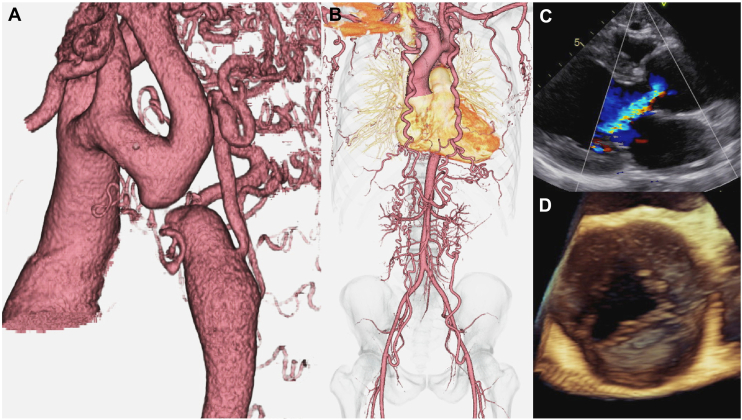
( A) Three-dimensional computed tomography shows loss of continuity of the aorta distal to the left subclavian artery bifurcation and ductus arteriosus and notably developed collateral circulation. (B) The brachial and left subclavian arteries are notably developed, with other collateral blood vessels to the lower limbs via the inferior abdominal wall. (C) Preoperative transthoracic echocardiography shows aortic root enlargement and severe aortic regurgitation. (D) Bicuspid aortic valve with raphe at the right and left coronary cusp identified by transesophageal echocardiography.
